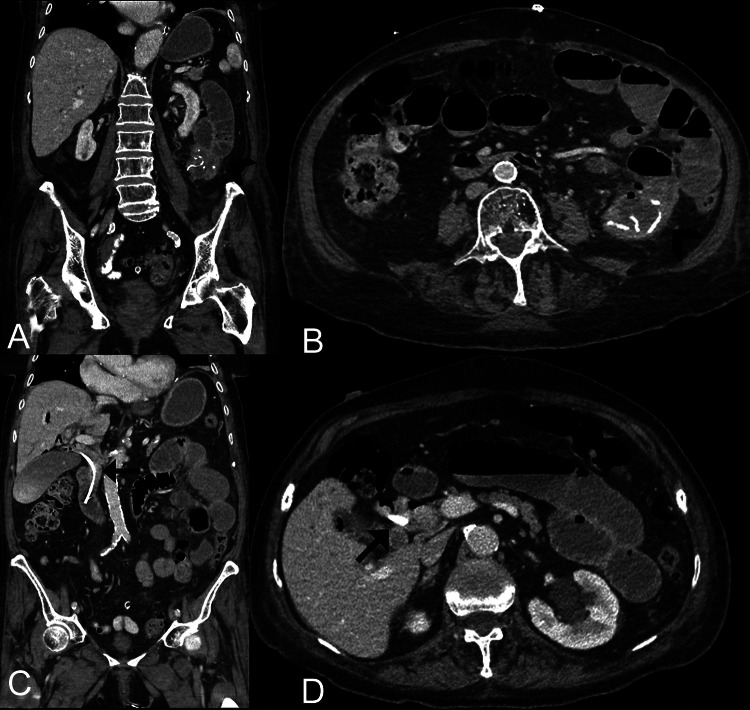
(A-D) (A and B) Contrast-enhanced follow-up CT following jejunum segment resection (black arrowhead) and after ERCP without signs of any postoperative complication. (c and d) depiction of the correct placed plastic endoprosthesis in the CBD (black arrows) after ERCP, although signs of subileus persist.
